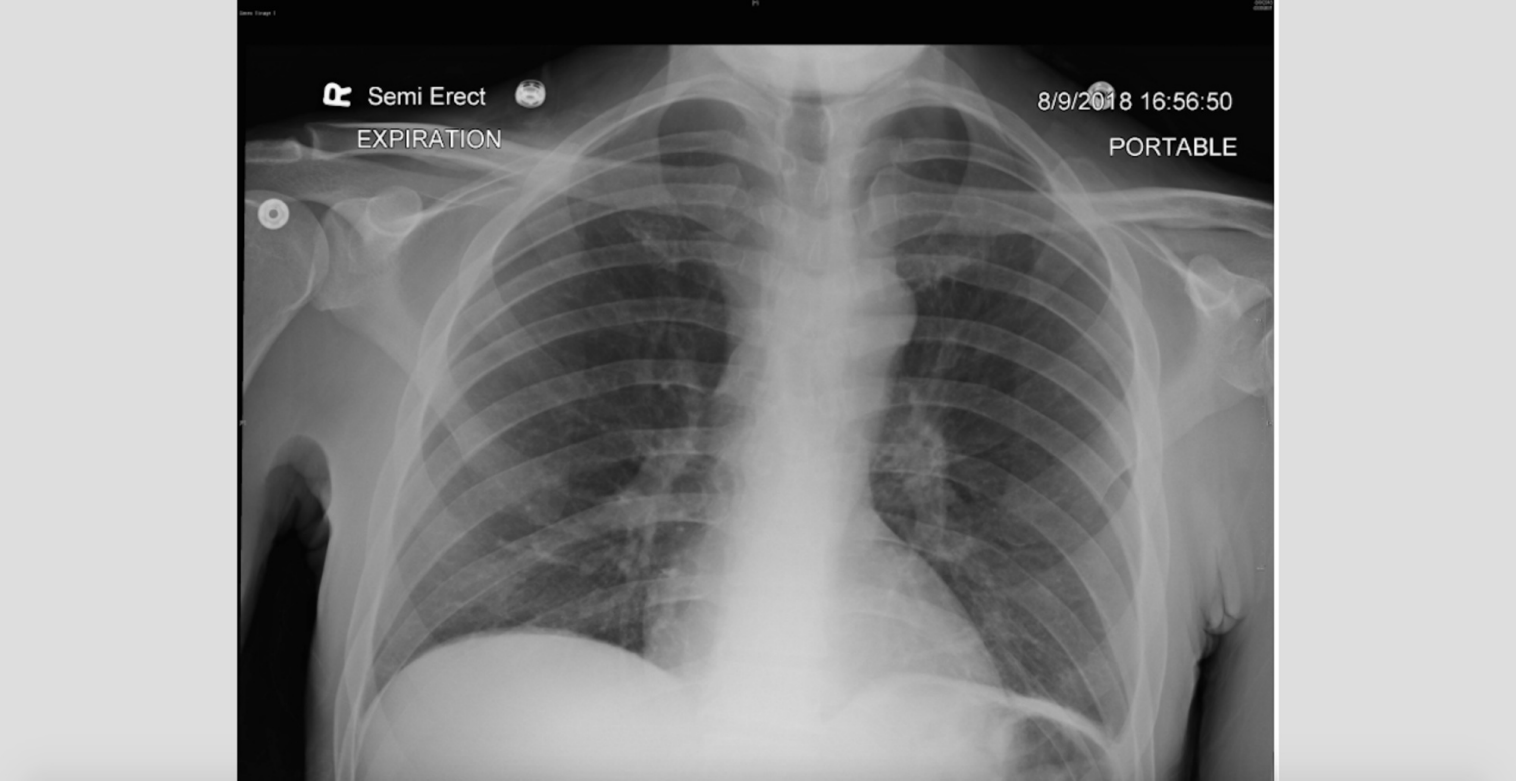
Repeat chest X-ray (CXR) on expiration showing likely spontaneous pneumothorax in the apical upper lobes.
